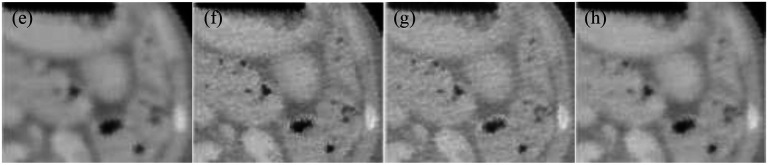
Comparison of CT image reconstructions from the Siemens scanner raw data of 576 projection views. (a) The image reconstructed using FBP, (b) the image reconstructed by SART with total variation, (c) the image reconstructed by score function-based method, (d) the image reconstructed by MSF reconstruction method, and (e–h) the enlarged image in the red rectangle in images (a–d), respectively.
